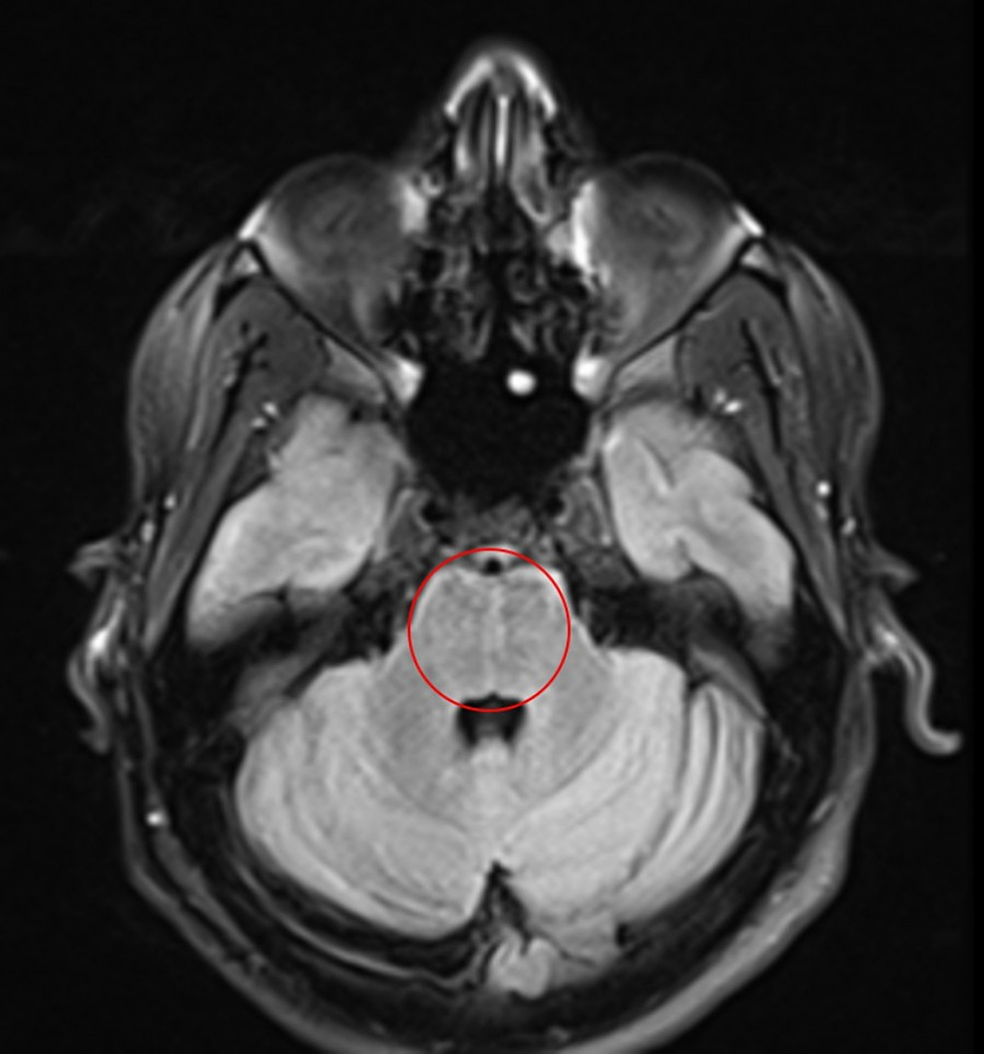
Axial MRI with FLAIR sequence showing signal resolution in the pons after administering pulse steroids (encircled). MRI: magnetic resonance imaging; FLAIR: fluid-attenuated inversion recovery
